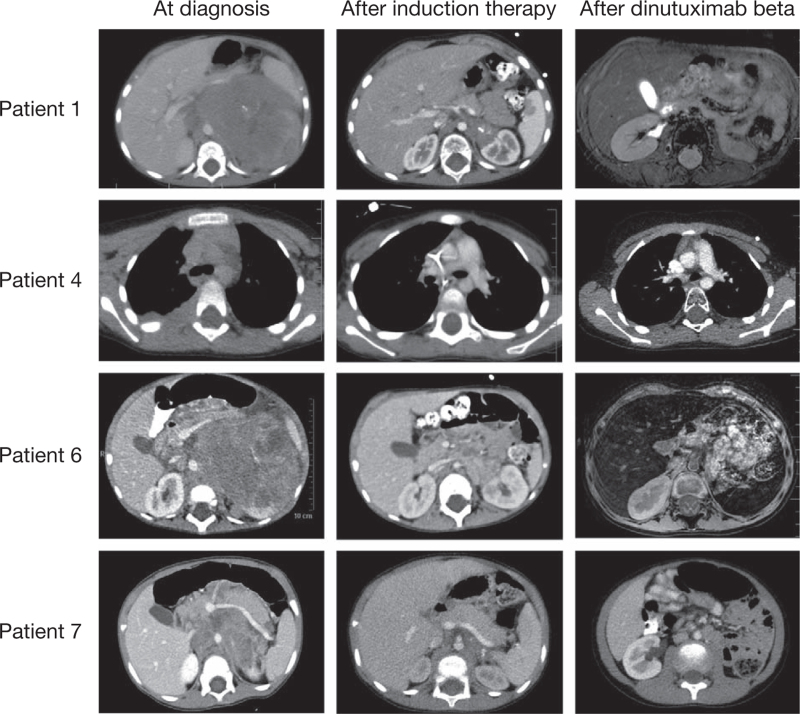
Abdominal/thorax CT or MRI scans at diagnosis (left panels), after induction therapy (middle panels) and after dinutuximab beta therapy (right panels) for patients who achieved a complete response with induction therapy: Patient 1, Patient 4, Patient 6, and Patient 7. CT = computed tomography, MRI = magnetic resonance imaging.
